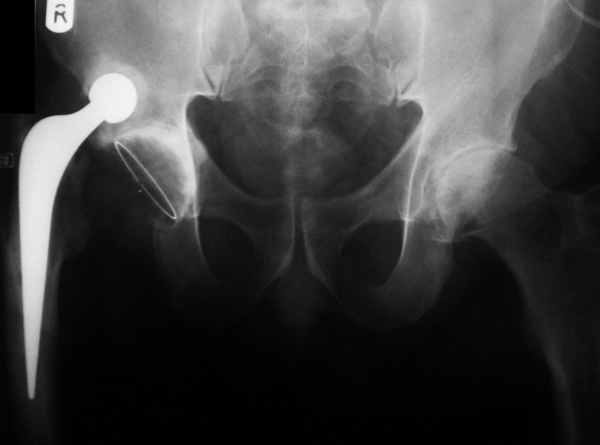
An anterioposterior radiograph of the pelvis showing degenerative changes of the left hip and the dislocated right Exeter total hip replacement, with the prosthetic femoral head articulating freely within a neoacetabulum.
